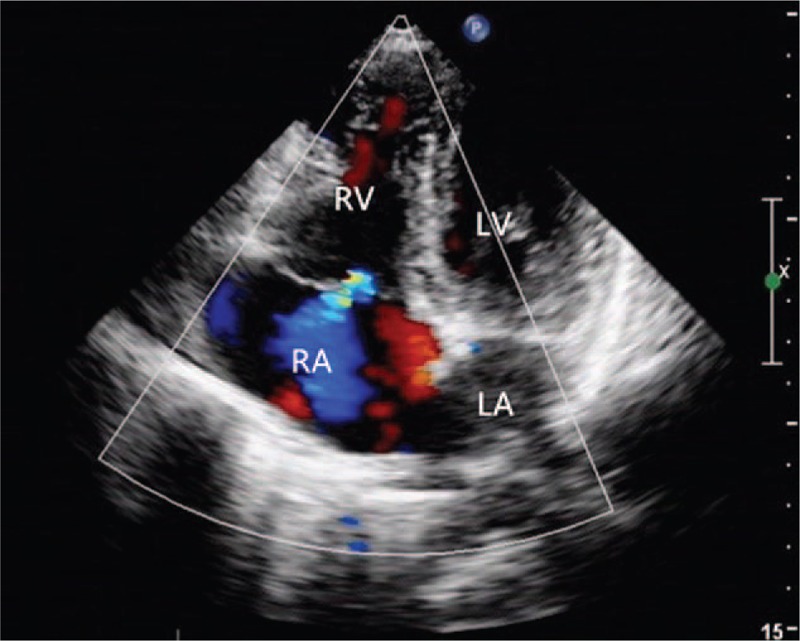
Echocardiographic image in the apical 4-chamber view showed slight of tricuspid regurgitation.
